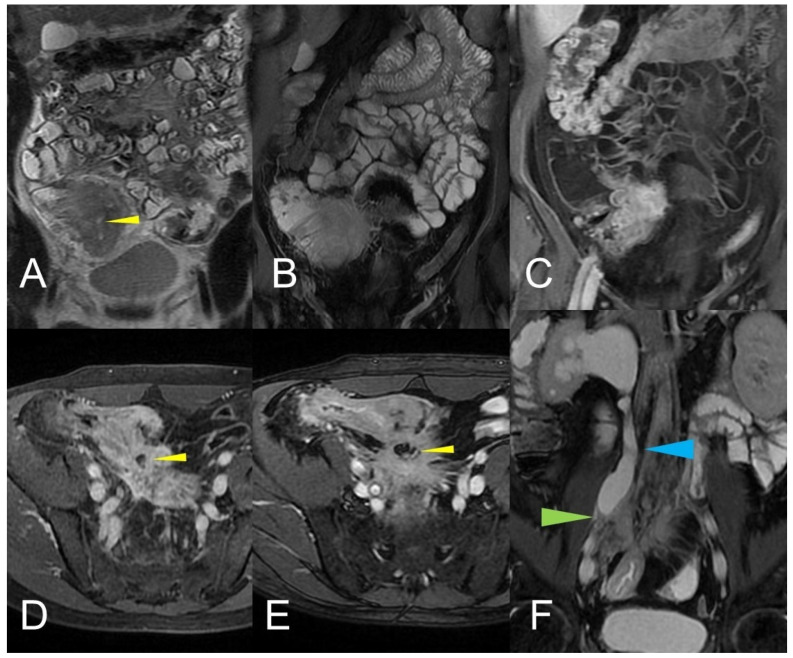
Large climbing inflammatory conglomerate. A small abscess is evident in the center of the inflammatory conglomerate (yellow arrowheads in (A,D,E)). It shows marked enhancement (yellow arrowhead in (C)) and determines conspicuous retracting effects both on the mesentery (spiculate aspect in (D,E)), in which it develops, and on the adjacent loops. In this patient, the right distal pelvic ureter is also narrowed, (green arrowhead in (F)) with hydronephrosis (blue arrowhead in (F)). (A): coronal T2-weighted MRE image; (B,F): coronal fast imaging employing steady-state acquisition (FIESTA) image; (C): coronal contrast-enhanced fat-suppressed T1-weighted image; (D,E): axial contrast-enhanced fat-suppressed T1-weighted image.
